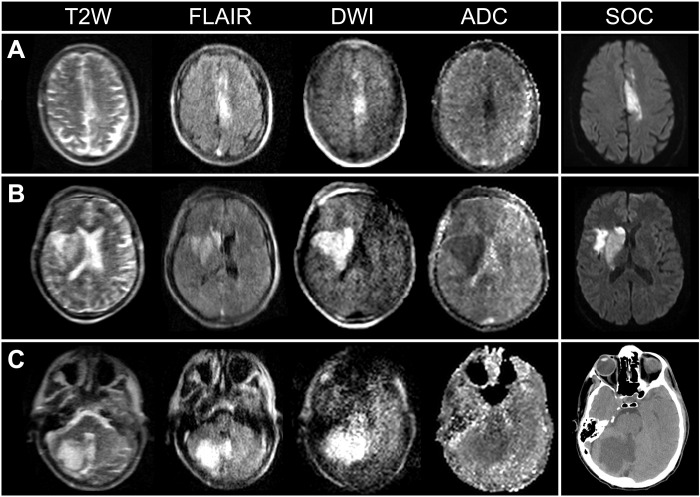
pMRI capturing ischemic infarcts throughout multiple regions of brain. (A) A 65-year-old male with a left anterior cerebral artery stroke imaged by pMRI and standard-of-care (SOC) MRI (1.5 T) (32 and 29 hours since last known normal, respectively). (B) A 61-year-old male with a right middle cerebral artery stroke imaged by pMRI and SOC MRI (1.5 T) (29 and 12 hours since last known normal, respectively). (C) A 57-year-old male with a right cerebellar stroke imaged by pMRI and SOC computed tomography (44 and 32 hours since last known normal, respectively). SOC exams included MRI (DWI sequence) and computed tomography.
